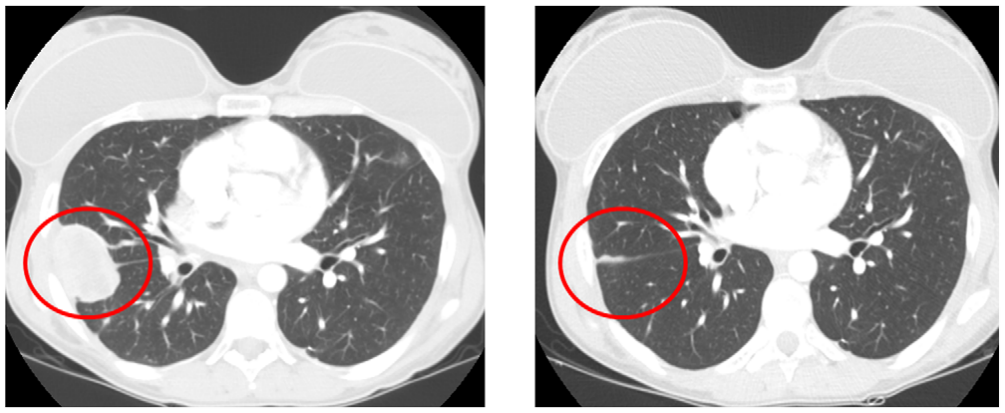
Imaging Responses in Ewing's sarcoma patient 1. CT of the thorax in patient 1 with Ewing's sarcoma response to IGF1R antibody (R1507) alone [12]. Left panel shows pre-treatment CT scan of the thorax showing metastatic Ewing's sarcoma in the lung. Right panel: Six weeks after IGF1R antibody (R1507) therapy shows regression of tumor.
